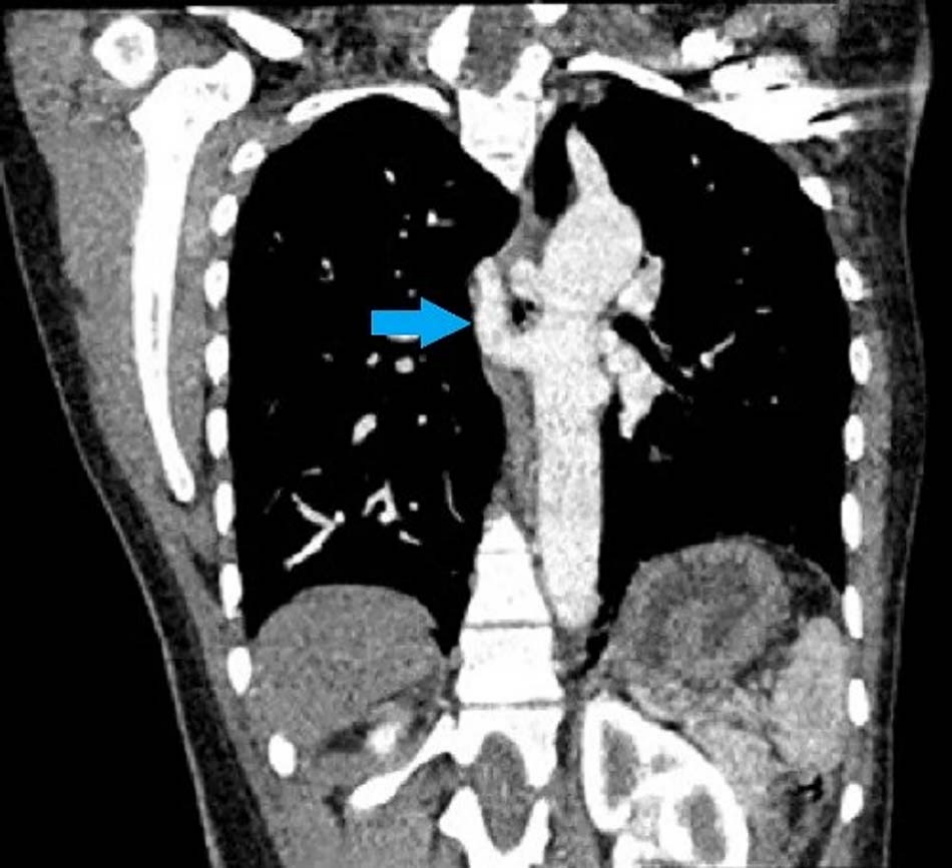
CT chest coronal view shows collaterals (blue arrow) originating separately from the descending aorta.
